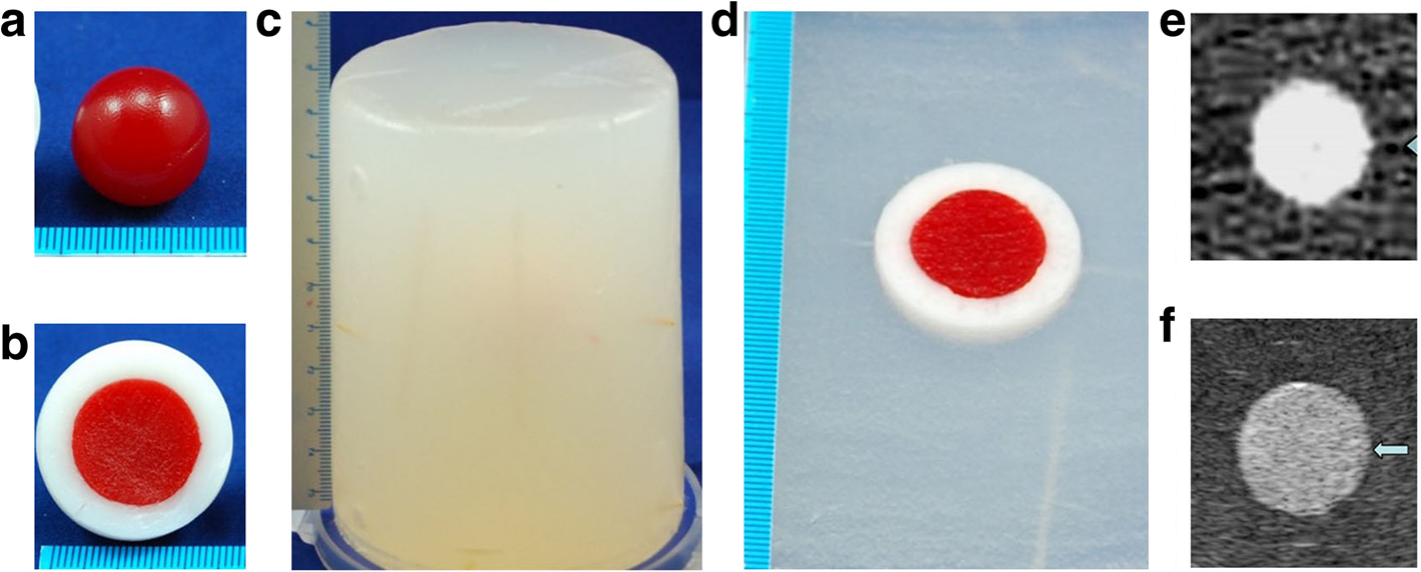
a A spherical tumor model (2 cm in diameter) made of carrageenan in red. b Section of the AM model: the carrageenan tumor model surrounded by the 5-mm AM gel in white. c Cylindrical-shaped parenchyma model (10 cm in height and 10 cm in diameter of the upper and lower plane). d Section of the cylindrical-shaped parenchyma model. e CT image showing the tumor. The scope of AM could not accurately evaluated. f US image showing the tumor. The boundary between the tumor and AM gel was not clear
